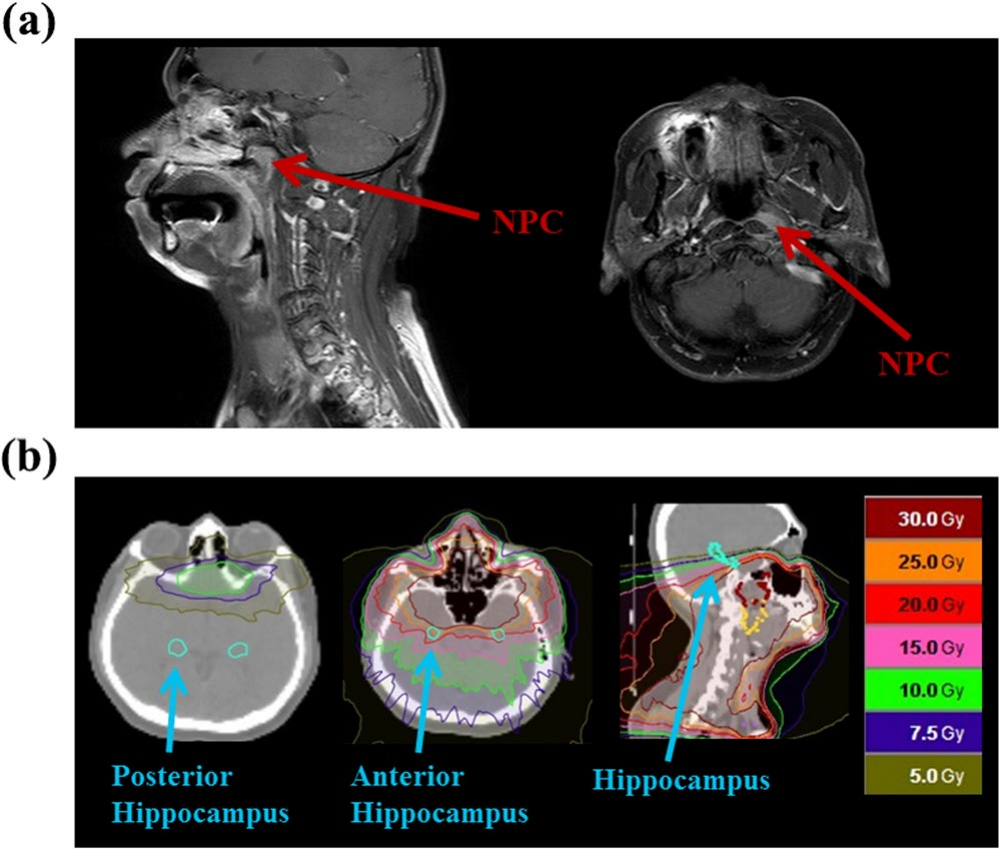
Dosimetric distribution in radiotherapy for nasopharyngeal carcinoma. (a) Sagittal and axial magnetic resonance imaging images were used to locate the tumor. (b) Overlay of an isodosimetric map on representative axial and sagittal computed tomography images showing the dose-dependent effect of radiation at the near temporal, frontal, and anterior hippocampal areas. The deposit dose at the hippocampus was 2–14 Gy, depending on the distance from the tumor.
